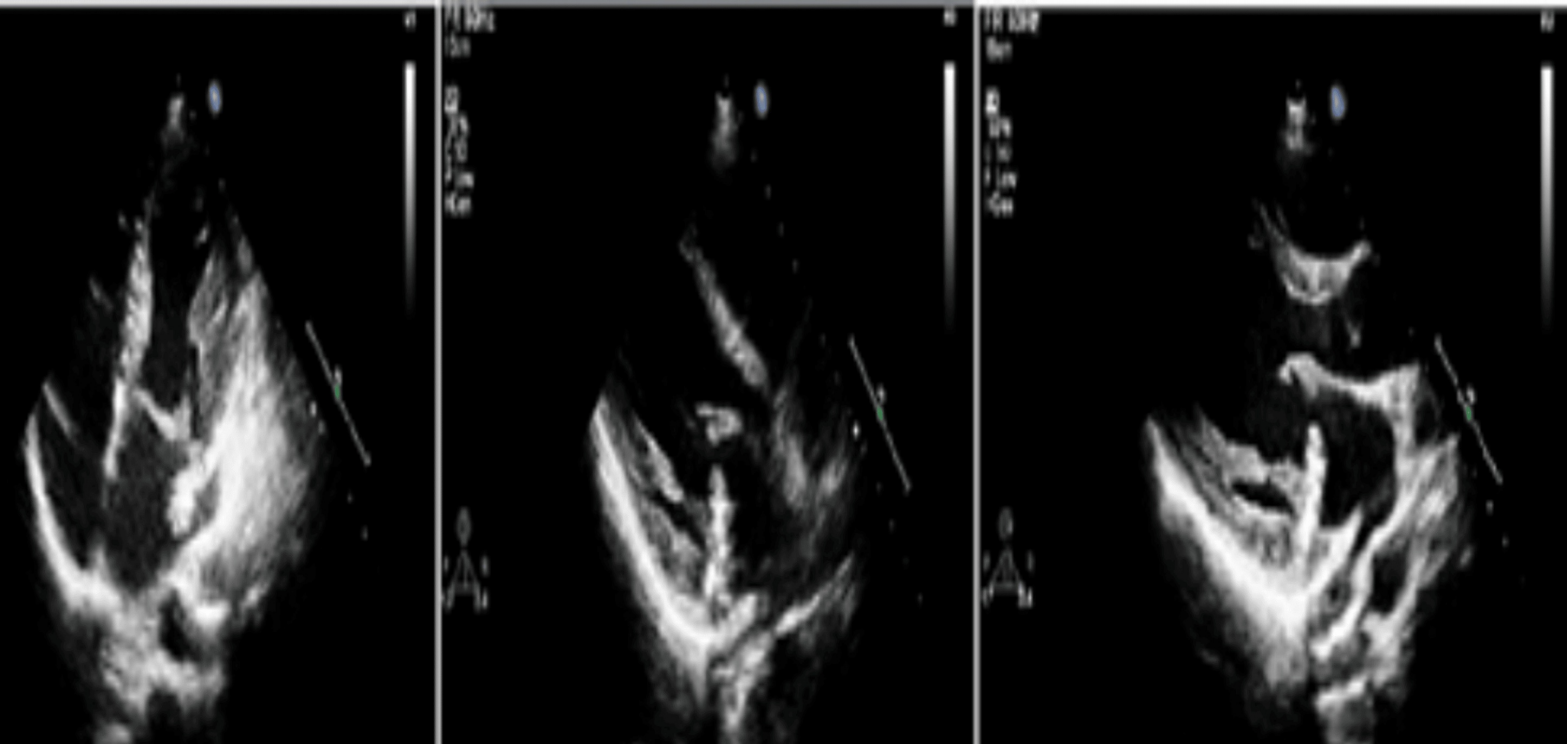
TTE examination The posterior cusp of the mitral valve was redundant, mobile, hypermobile, measuring 15-16 mm in length and 6-7 mm in width, prolapsing without restriction. A mass-like image compatible with vegetation was observed on its atrial surface. Systolic annular dysfunction of approximately 12 mm was noted in the mitral annulus lateral region TTE: transthoracic echocardiography
